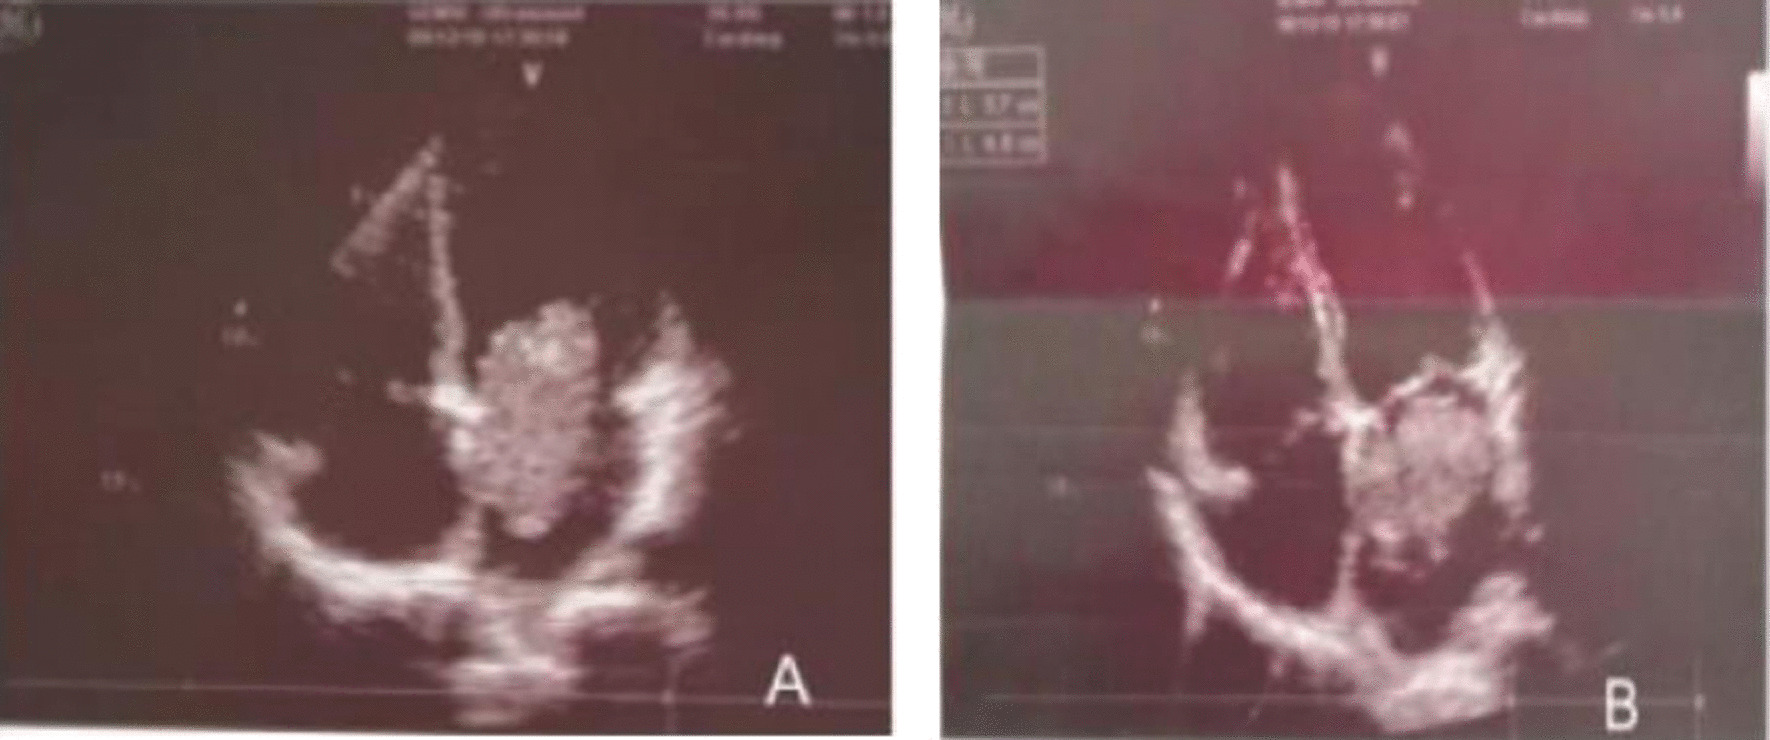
Preoperative transthoracic echocardiography (TTE) showing a pedunculated atrial heart mass (37 × 48mm) on the interatrial septum, protruding through the mitral valve (A) and for the case 2 (B), a large mass (64X26mm) attached to the roof of the left atrium
